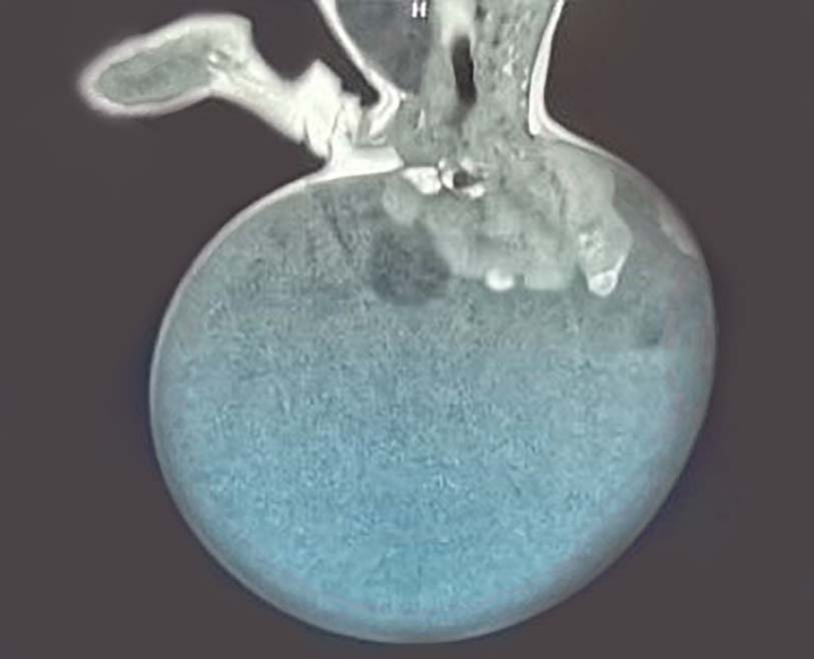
Magnetic resonance imaging showed a complete external component.
